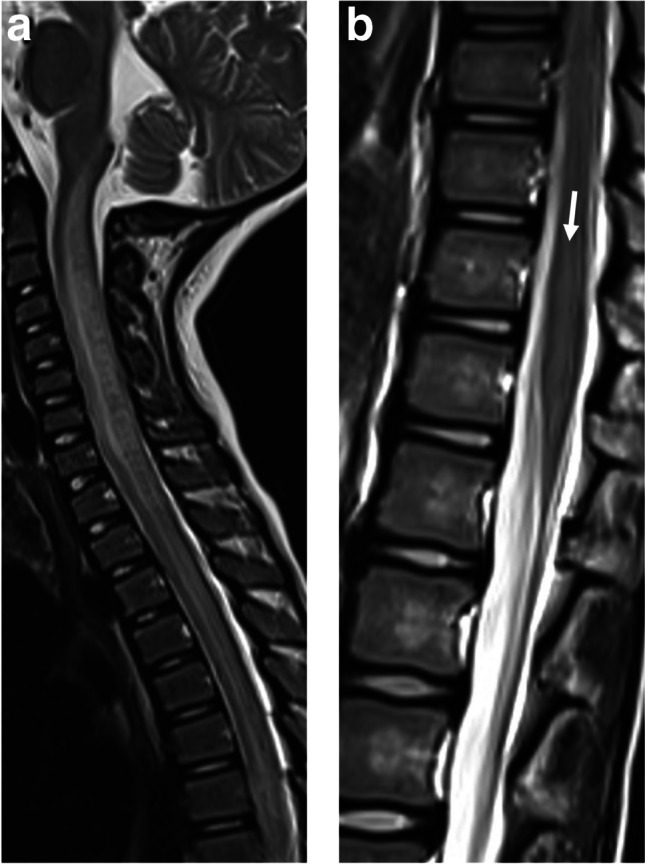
Transverse myelitis in myelin oligodendrocyte glycoprotein antibody-associated disease. a Sagittal T2-weighted magnetic resonance (MR) image obtained from a 5-year-old girl shows a longitudinally extensive hyperintensity with swelling along the cervical and upper thoracic cord, extending superiorly to the level of the area postrema. b Sagittal T2-weighted MR image obtained from of a 7-year-old girl shows a longitudinally extensive hyperintensity along the lumbar cord, with typical conus involvement (*arrow*)
